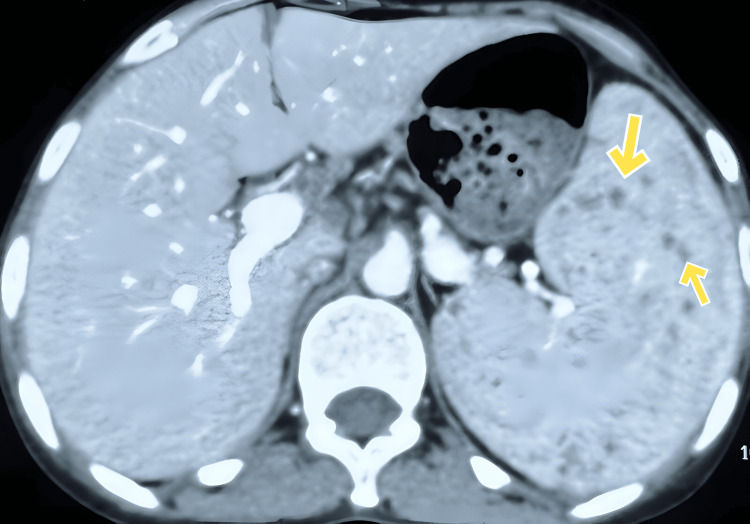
CT image showing the presence of multiple micro-abscesses in the spleen
